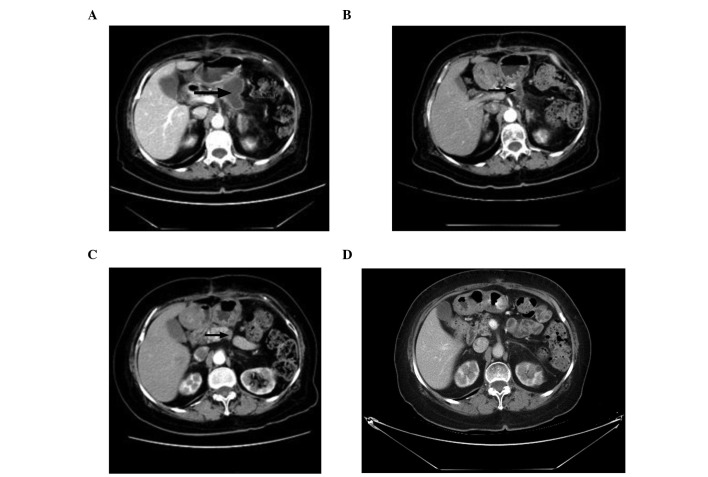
(A) November 10, 2010: Postoperative arterial phase contrast-enhanced computed tomography (CT) scan showing a low-density nodule. (B) February 14, 2011: Following 4 cycles of CIK cell infusion, the arterial phase contrast-enhanced CT scan shows that the low-density nodule has markedly decreased in size. (C) July 11, 2011: Following 12 cycles of CIK cell infusion, the arterial phase contrast-enhanced CT scan reveals that the low-density nodule has slighlty decreased in size. (D) February 29, 2012: The CT scan shows that the low-density nodule has almost completely disappeared.
